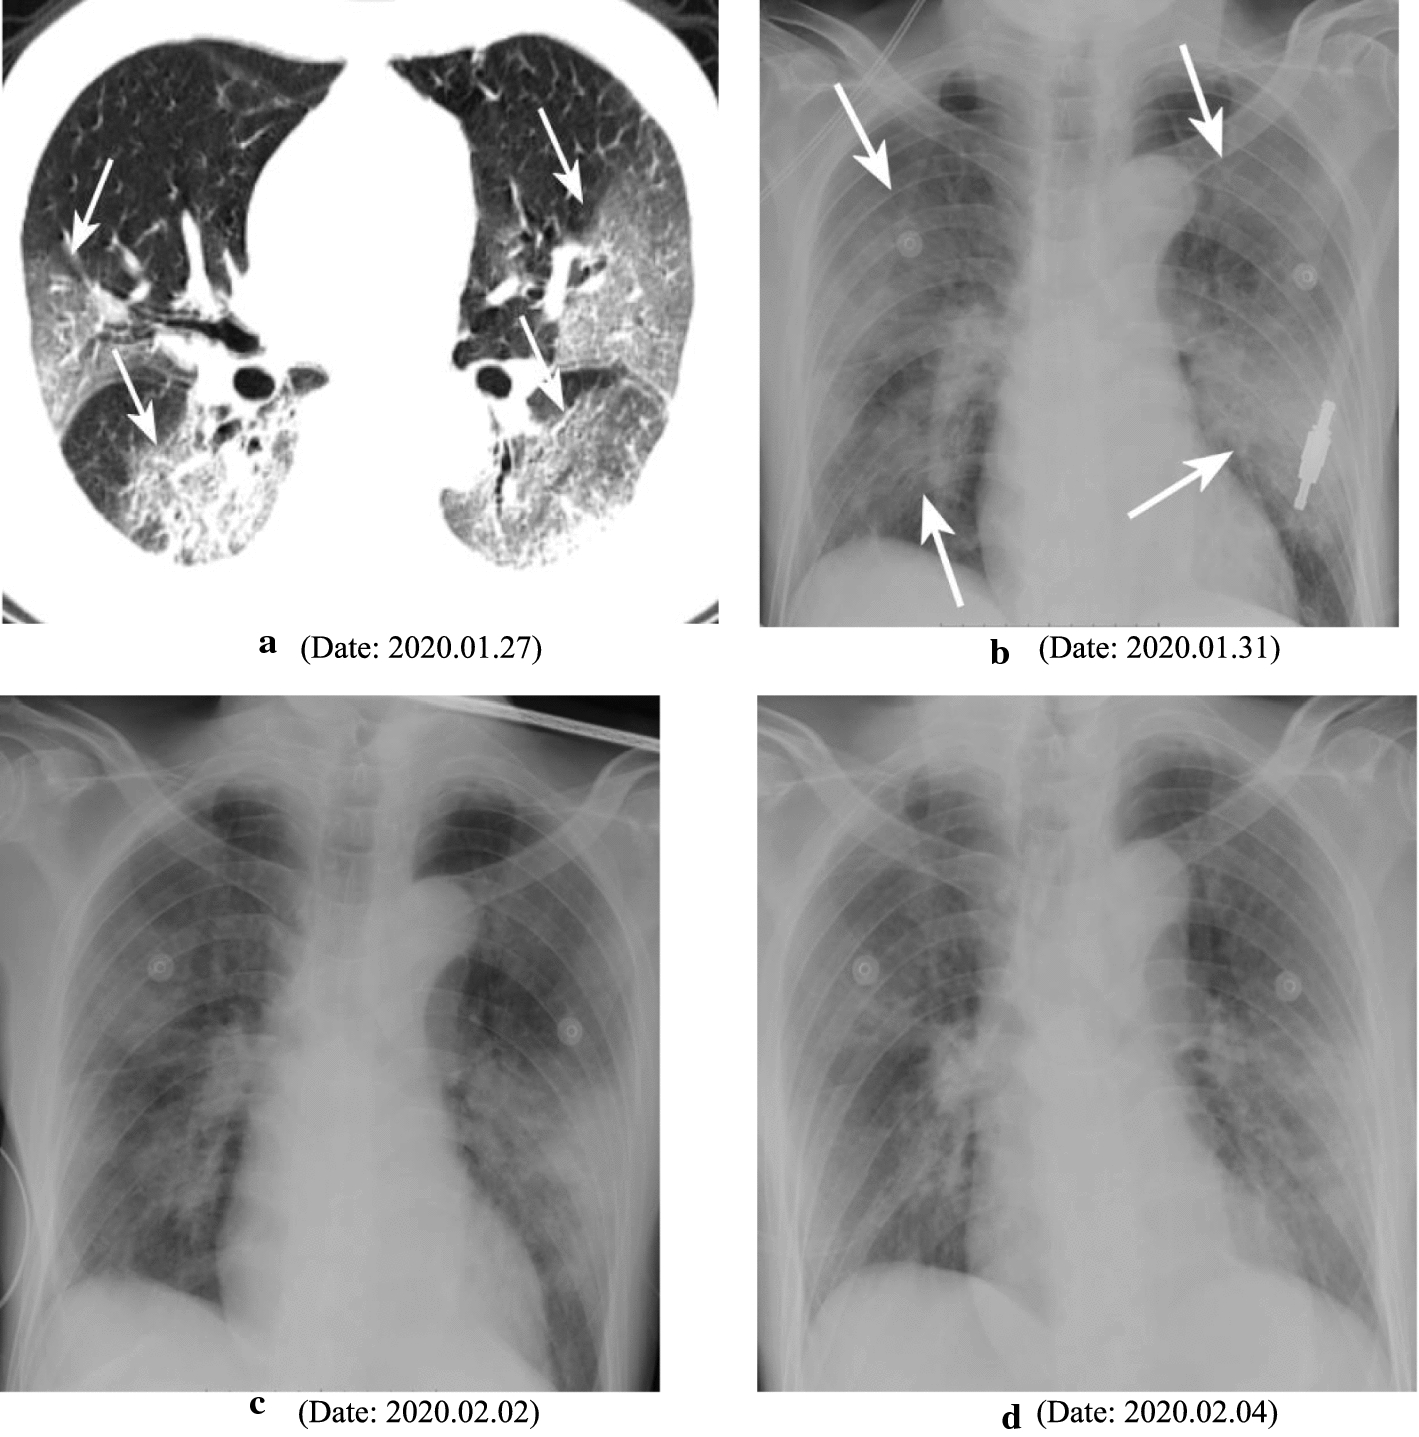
CT and DR images of a 76-year-old male with fever, cough and expectoration: a Chest CT scan. b–d Follow-up DR images
